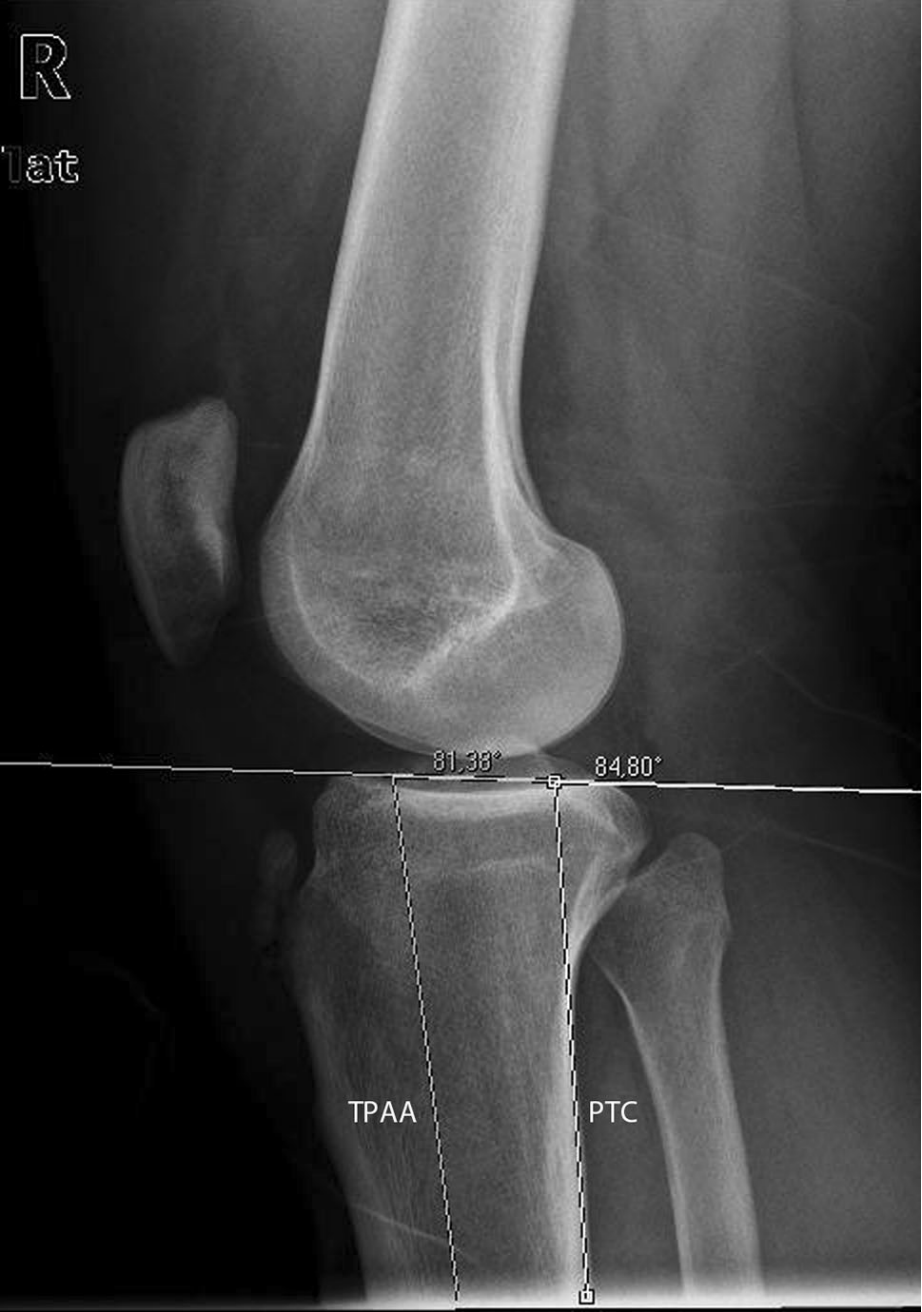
On a true lateral-view radiograph on the knee, the angle between the tangent to the medial tibial plateau and the perpendicular direction to the tibial proximal anatomical axis (TPAA) and posterior tibial cortex (PTC) were measured
